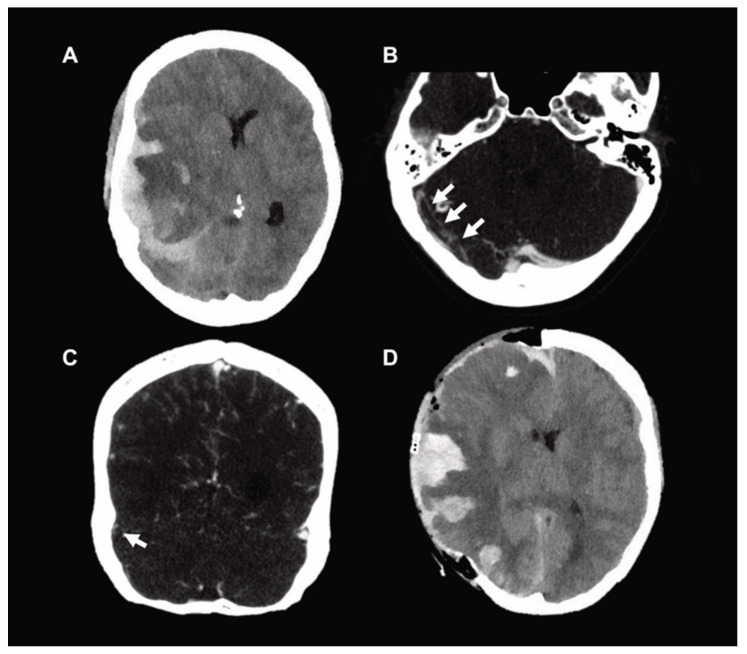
(A) Preoperative CT scan which demonstrates parenchymal stasis hemorrhage and a spontaneous acute subdural hematoma with associated midline shift and impending herniation. (B,C) Axial and coronal images, respectively, which demonstrate marked sinus vein thrombosis (arrows) on CT venography (CTV). (D) Postoperative CT scan after decompressive craniectomy.
